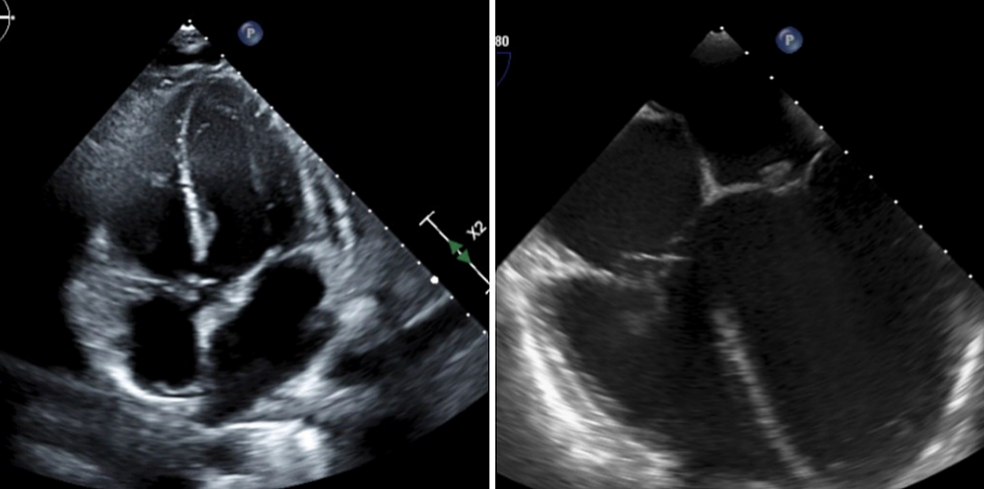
TTE and TEE showing mitral and tricuspid valve vegetations. The left-sided image showed a transthoracic echocardiogram (TTE) and the right-sided image a transesophageal echocardiogram (TEE).
